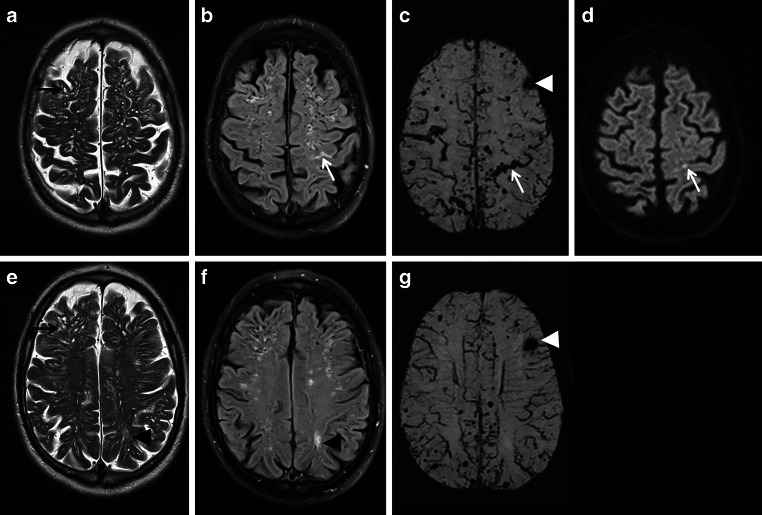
A 72-year-old man with beginning dementia suffering from temporary hemiparesis right and aphasia due to cerebral amyloid angiopathy (CAA). Acute cortical subarachnoid hemorrhage (cSAH); b fluid attenuated inversion recovery [FLAIR ax.; c susceptibility-weighted imaging (SWI) ax.; d diffusion-weighted imaging (DWI) ax., b = 1000 s/mm^2^, *arrow*. Enlarged perivascular spaces (PVS) (a,e: T2WI ax.; *arrow*), focal small gliosis (b,f: FLAIR ax.; *arrowhead*), multiple microbleeds (MB) and cortical superficial siderosis (cSS) (c,g: SWI ax.) beside residual atypical intracerebral bleeding frontal left (c,g: *arrowhead*)
